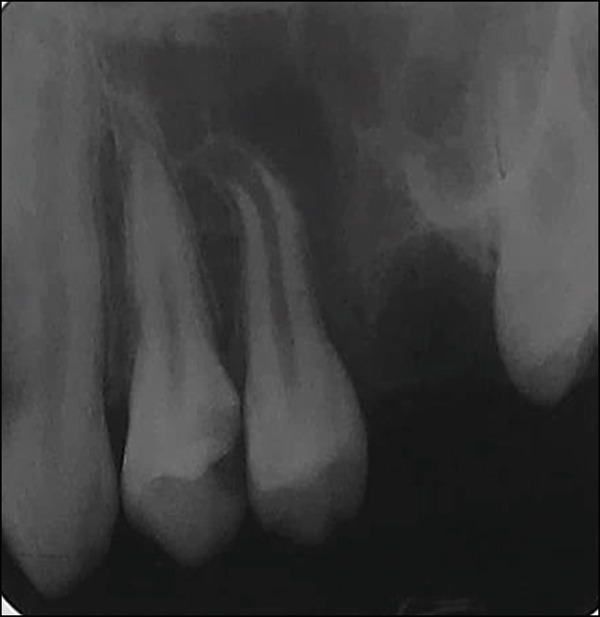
Preoperative periapical radiograph of 24.
